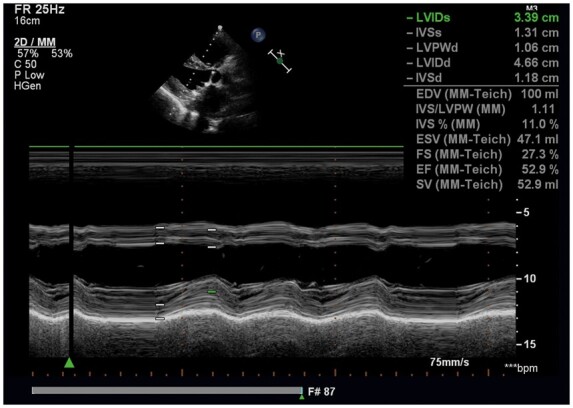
Transthoracic echocardiography showing normal left ventricular function.
